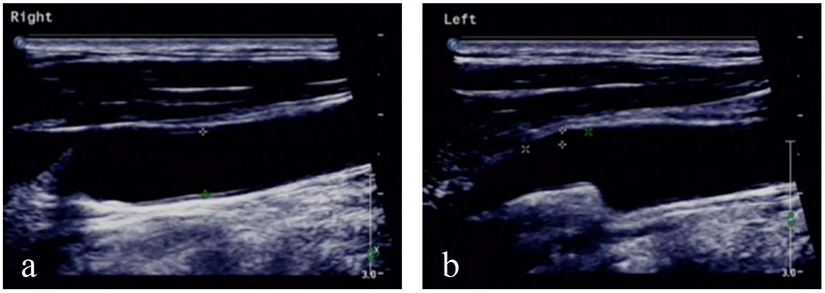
Ultrasound of neck vessels. (a) Ultrasound of the right common carotid artery showed a diameter of 0.78 cm and an intima-media thickness of 0.10 cm. (b) Left common carotid artery ultrasound showed a diameter of 0.75 cm, an intima-media thickness of 0.10 cm, and a hypoechoic flat plaque (2.00 × 0.37 cm) on the distal posterior wall.
